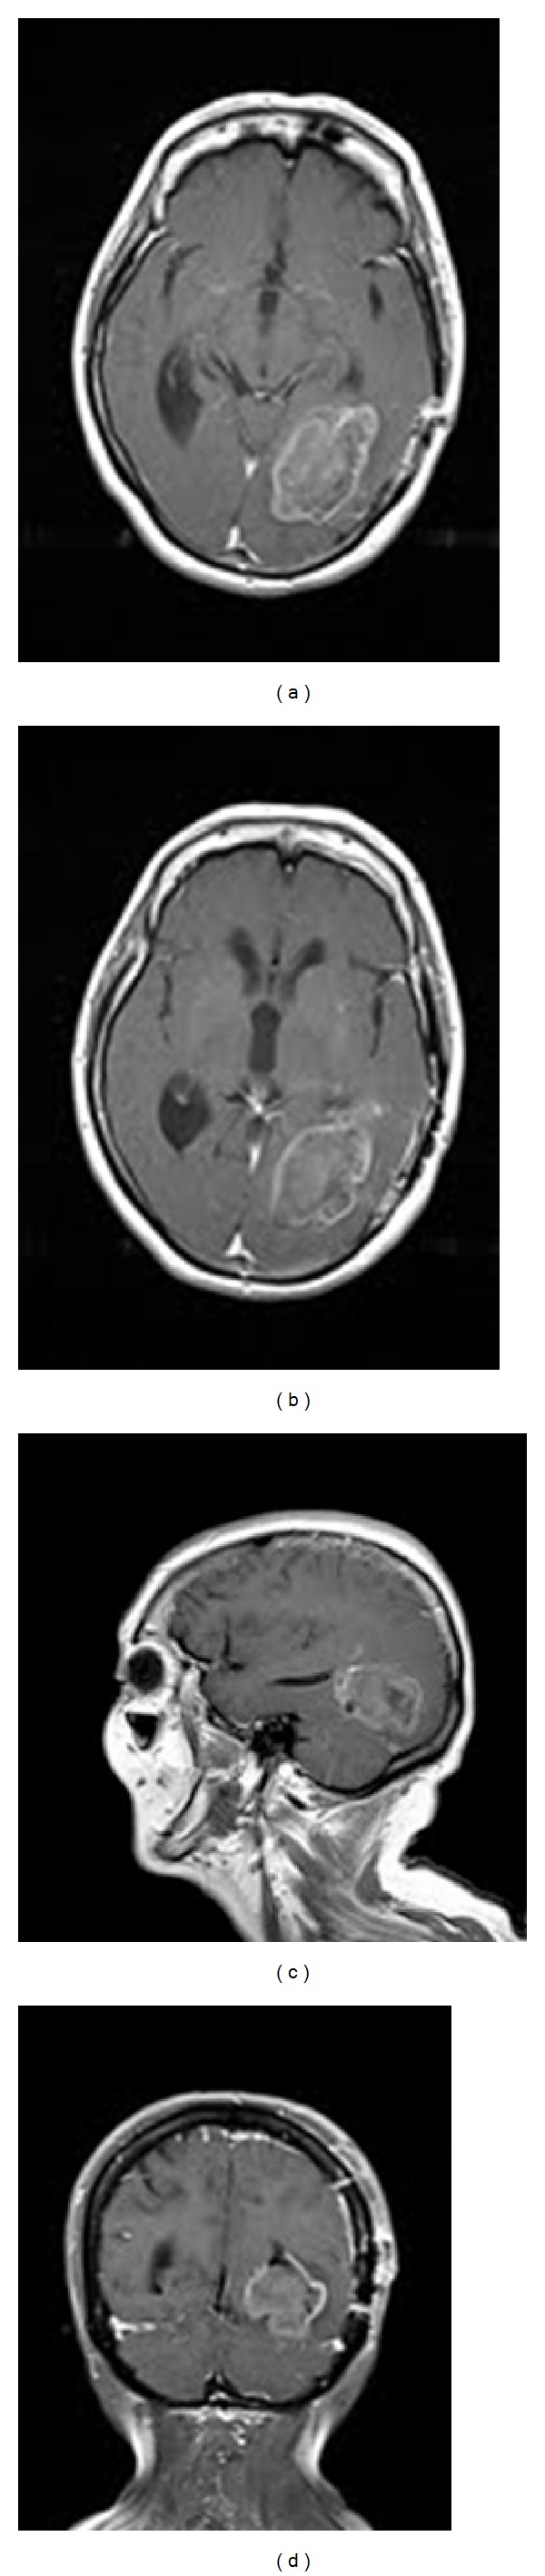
Brain MRI at second admission. (a), (b) Axial T1-weighted image with gadolinium, showing a larger mass with peripheral enhancement compared to the original appearance of the lesion as seen in Figure 1. (c) Sagittal T1-weighted image with gadolinium, showing the larger lesion with a more substantial intraparenchymal component. (d) Coronal T1-weighted image with gadolinium also shows the enlarged lesion with greater mass effect.
